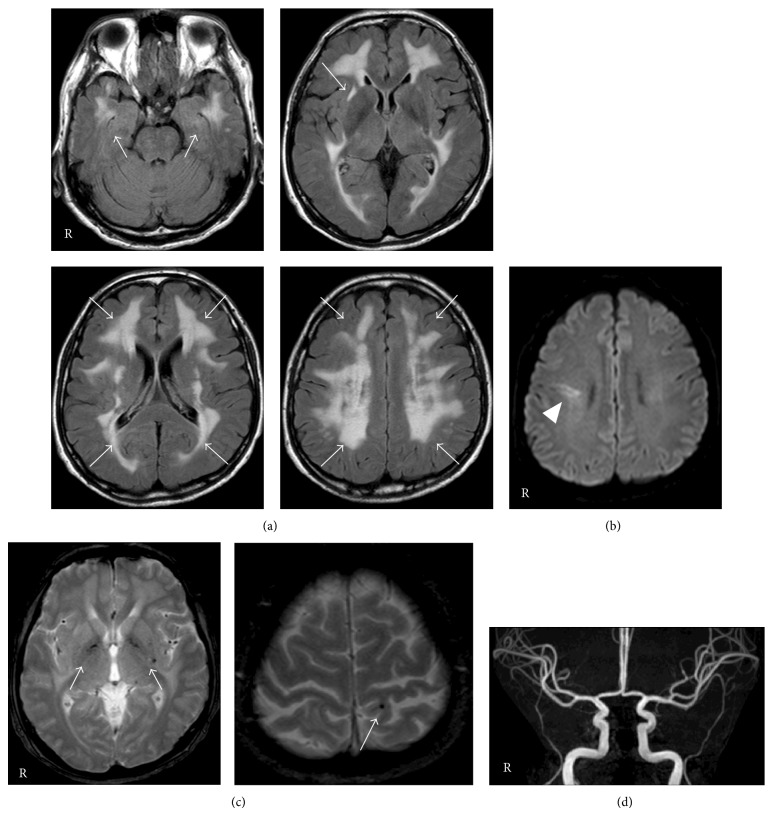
Magnetic resonance images and magnetic resonance angiograms. (a) Fluid attenuated inversion recovery (FLAIR) images demonstrated diffuse hyperintensities in the periventricular and subcortical white matter involving both temporal pole regions and the external capsule (arrow). (b) A hyperintense area on diffusion-weighted images (arrowhead). (c) T2^*^-weighted images showed multiple microbleeds (arrow). (d) Magnetic resonance angiograms.
